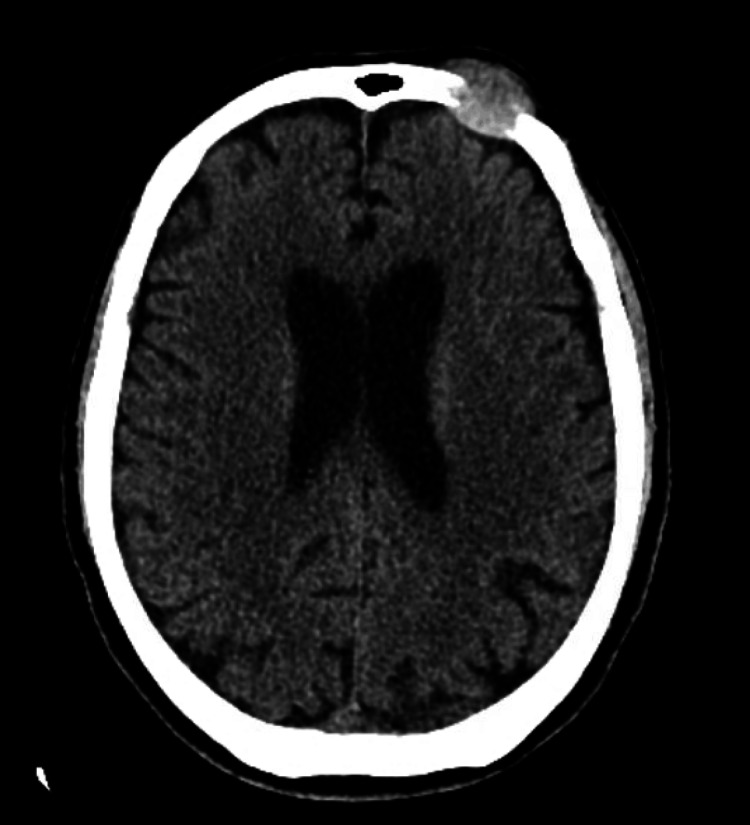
CT of the head without contrast. There is a 2.8x1.7x2.5 cm mass in the anterior left frontal skull with bony destruction, suggesting a neoplasm/metastasis. There is a mild mass effect on the anterior left frontal cortex due to the left frontal skull mass. There is no midline shift.
